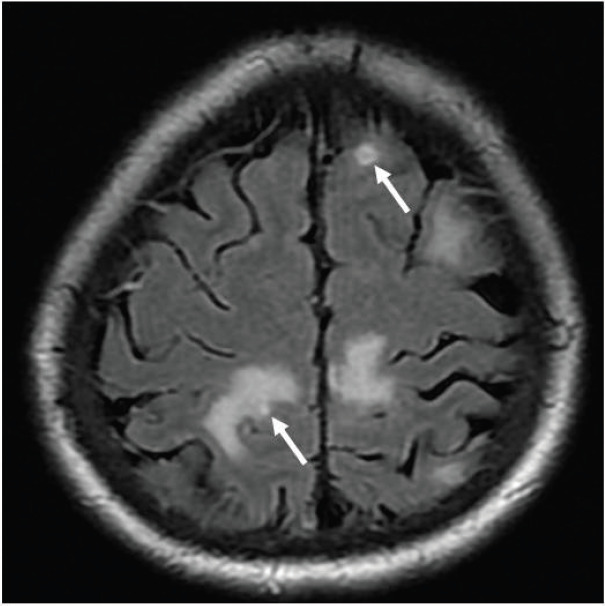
Axial T2-FLAIR post contrast-enhanced image showing other lesions within the bilateral grey white junction, some with focal enhancement (thick arrows).
